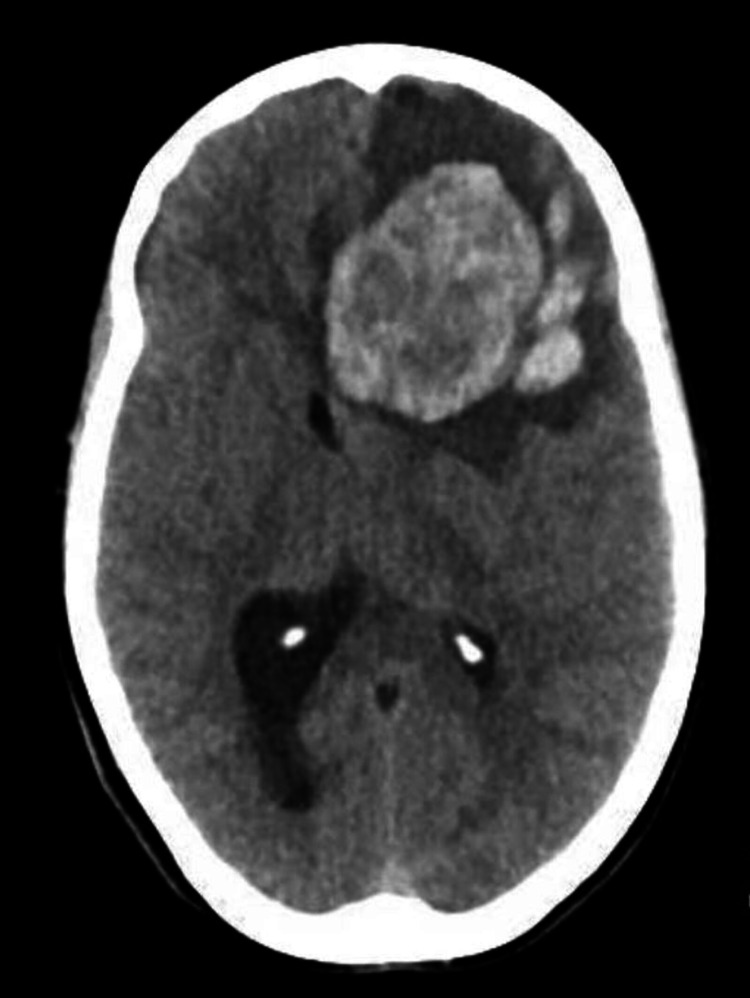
Noncontrast head CT in axial plane preceding surgical decompression showing a large left frontal lobe mass measuring 4.5 x 5.2 cm with anterior and inferior acute intraparenchymal hemorrhage and significant surrounding edema and mass effect.
